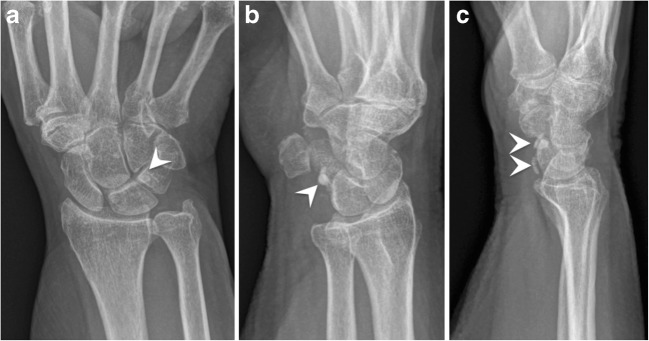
Periarticular calcifications following corticoid injection. (a) Frontal, (b) oblique and (c) lateral radiographs of the wrist show palmar soft tissue calcifications (arrowhead) following carpal tunnel injection of corticoid
